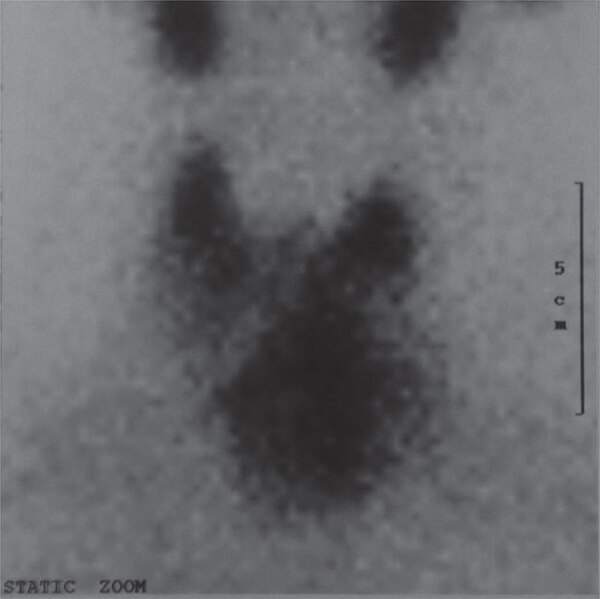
Pre-operative 99mTc scintigraphy (uptake 2.4%).
